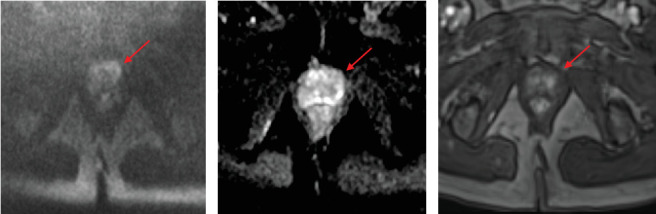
Representative MRI of left apex anterior lesion (red arrows). (a) DWI, (b) ADC, and (c) dynamic postcontrast. There is a subtle, asymmetric, increased signal noted retrospectively on DWI that corresponds to the lesion demonstrating hypermetabolic activity on PSMA PET/CT. There is a very mild hypointense signal on ADC with asymmetric early enhancement on postcontrast images. This lesion was assigned a PI-RADS score of 4.
